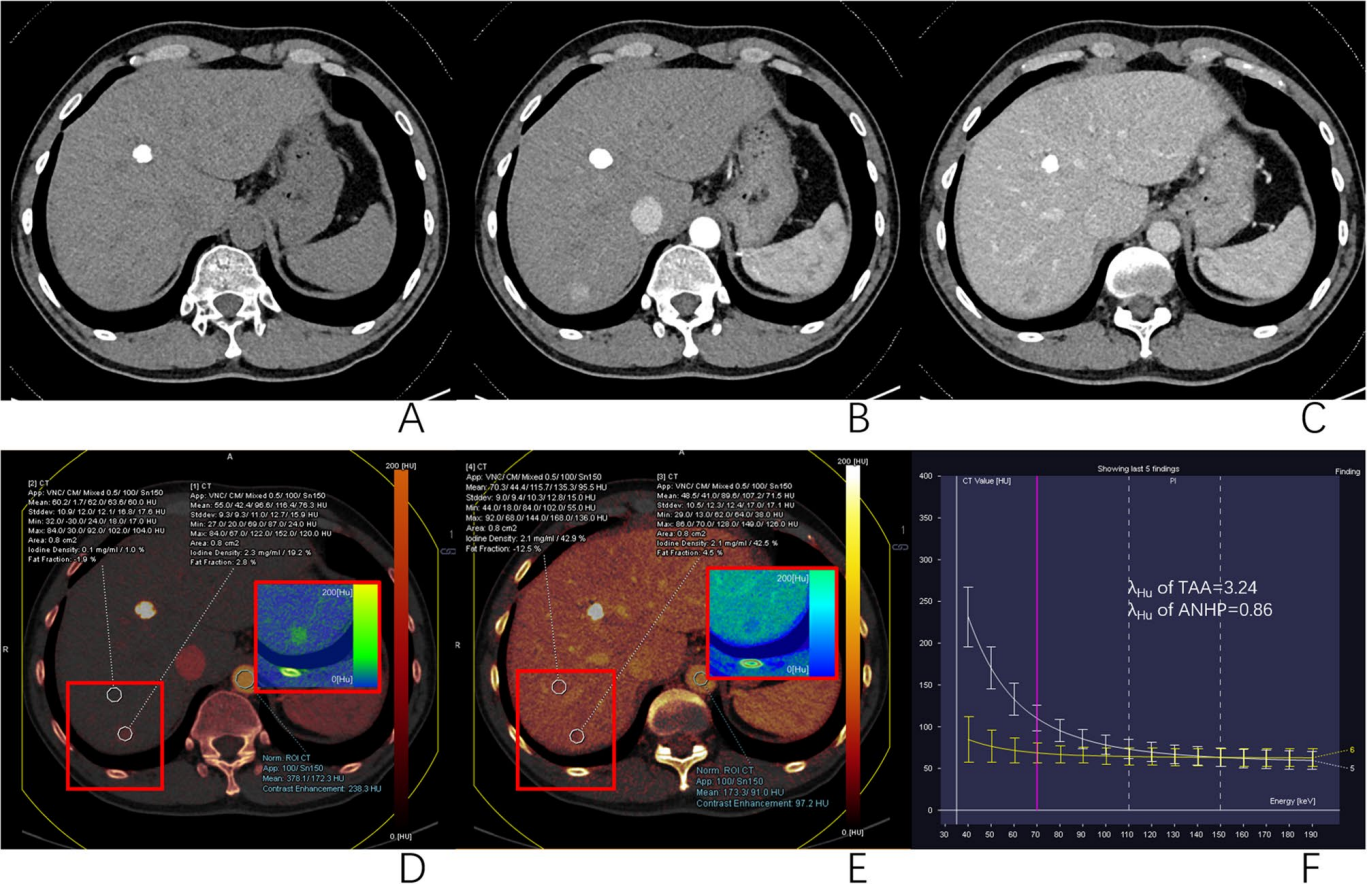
DECT images of a 52-year-old man with HCC undergoing lipiodol TACE. The right hepatic lobe showed the original lesions were completely deposited with lipiodol, and the new lesions appeared, a low-attenuation nodule on axial unenhanced CT image (A), significant enhancement at the arterial phase (B), and wash-out at the portal venous phase(C). ROIs of TAA and ANHP (D and E) placed on arterial and portal iodine map showed IC and NIC of TAA to be 2.3 mg/ml and 19.2% at arterial phase, and 2.1 mg/ml and 42.5% at portal venous phase. IC and NIC of ANHP was 0.1 mg/ml and 1.0% at arterial phase, and 2.1 mg/ml and 42.9% at portal venous phase. The λ_Hu_ of TAA and ANHP was 3.24 and 0.86, respectively (F).
